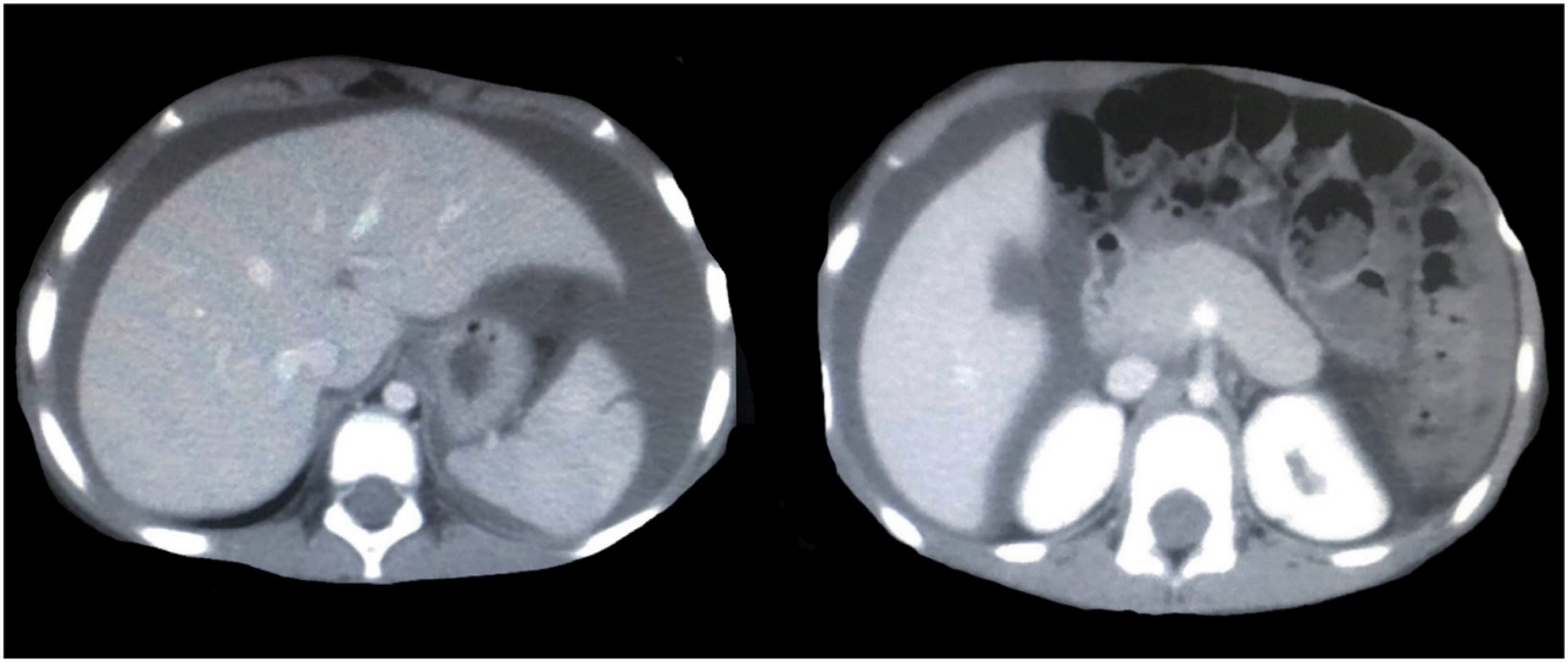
CT abdominopelvic report: evidence of severe free fluid is seen in the abdominopelvic cavity.
